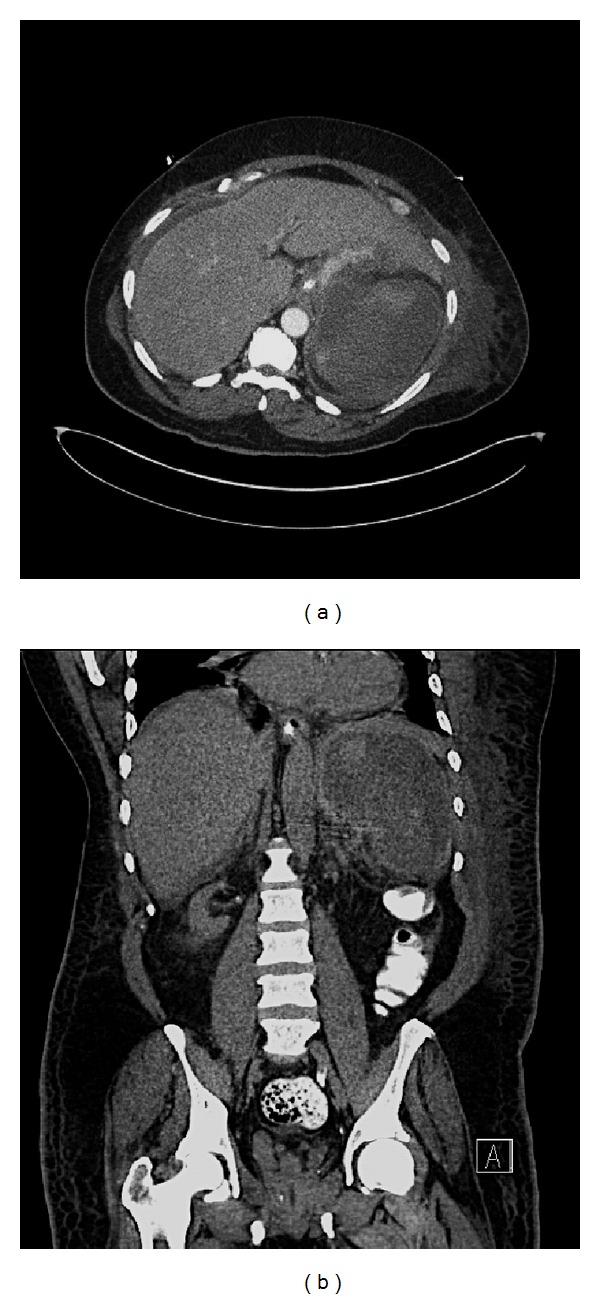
Contrast-enhanced computed tomographic images of the abdomen show massive splenic infarction evidenced by a diffusely hypodense spleen at presentation to emergency department.
